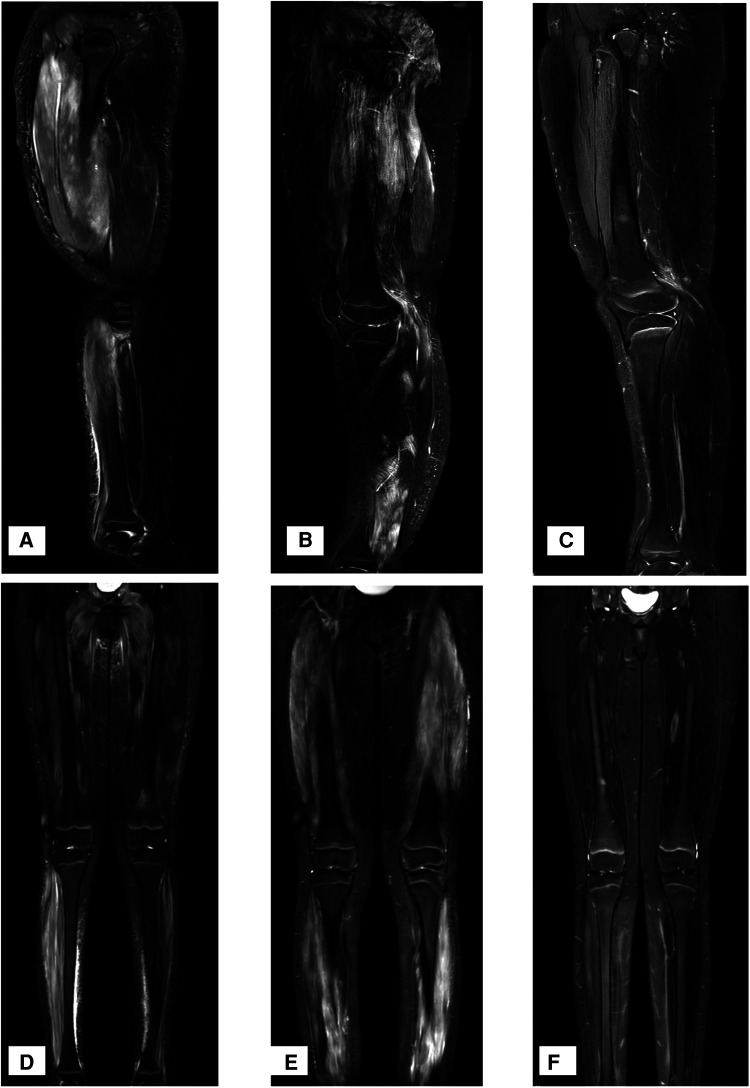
MRI T2W and stir-weighted images of the muscles at the time of onset. (A,D) T2W and Stir images at the time of onset, showing a high signal on T2-weighted and Stir images. (B,E) T2W and Stir images at the time of recurrence, showing extensive muscle damage. (C,F) T2W and Stir images one year after the application of Adalimumab.
